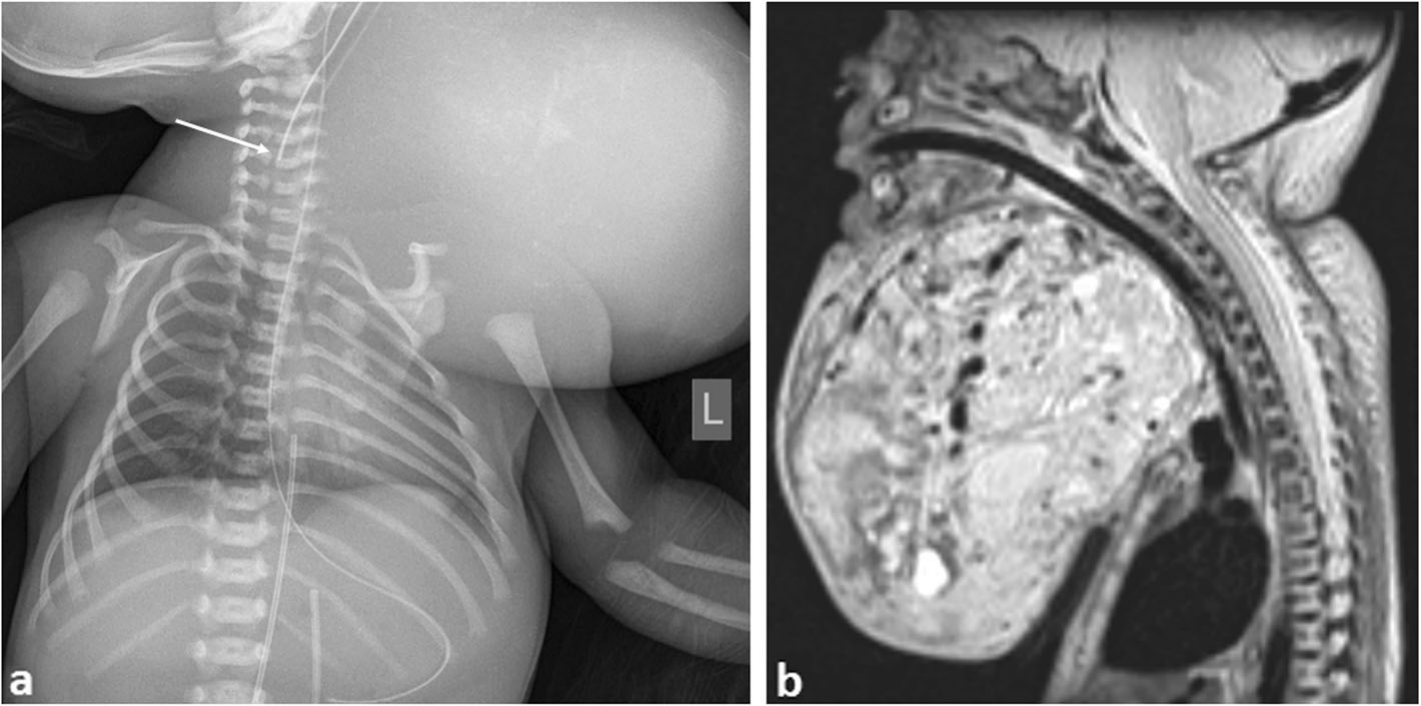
A neonate antenatally diagnosed with a large cervical teratoma. The patient was delivered at 34 weeks gestation by ex utero intrapartum treatment (EXIT) procedure due to impending airway obstruction. a Initial chest radiograph of patient post intubation. Note the high position of the endotracheal tube tip from difficult intubation (arrow). This should lie at the T2 vertebral level. b Sagittal postnatal T2-weighted MRI image demonstrating the large cervical mass and proximity to the upper airway. The patient had been successfully intubated during the MRI study, thus accounting for the patency of upper airway diameter in this image
